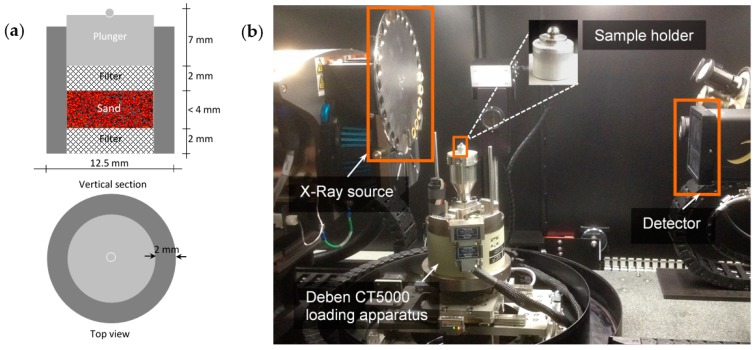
(a) Sample holder setup; (b) Test setup within X-ray computed tomography (X-ray CT) machine.
